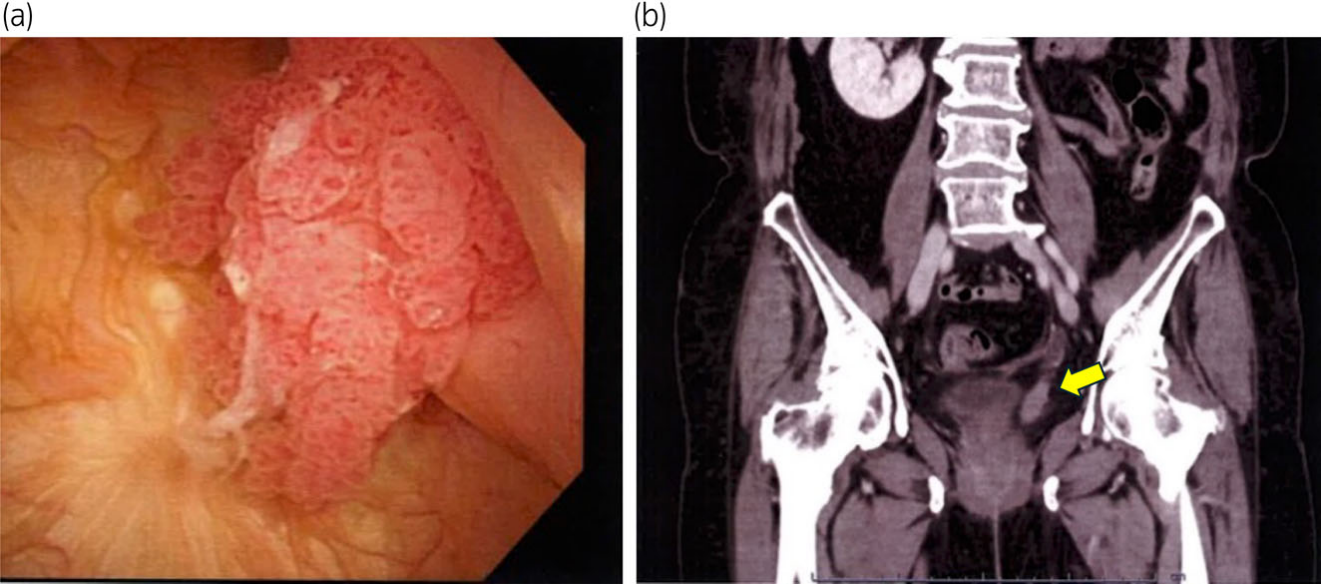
(a) A papillary tumor exiting the left ureteral orifice is seen on cystoscopy. (b) Tumor filling in the remaining ureter in the coronal view on CT (yellow arrow).
